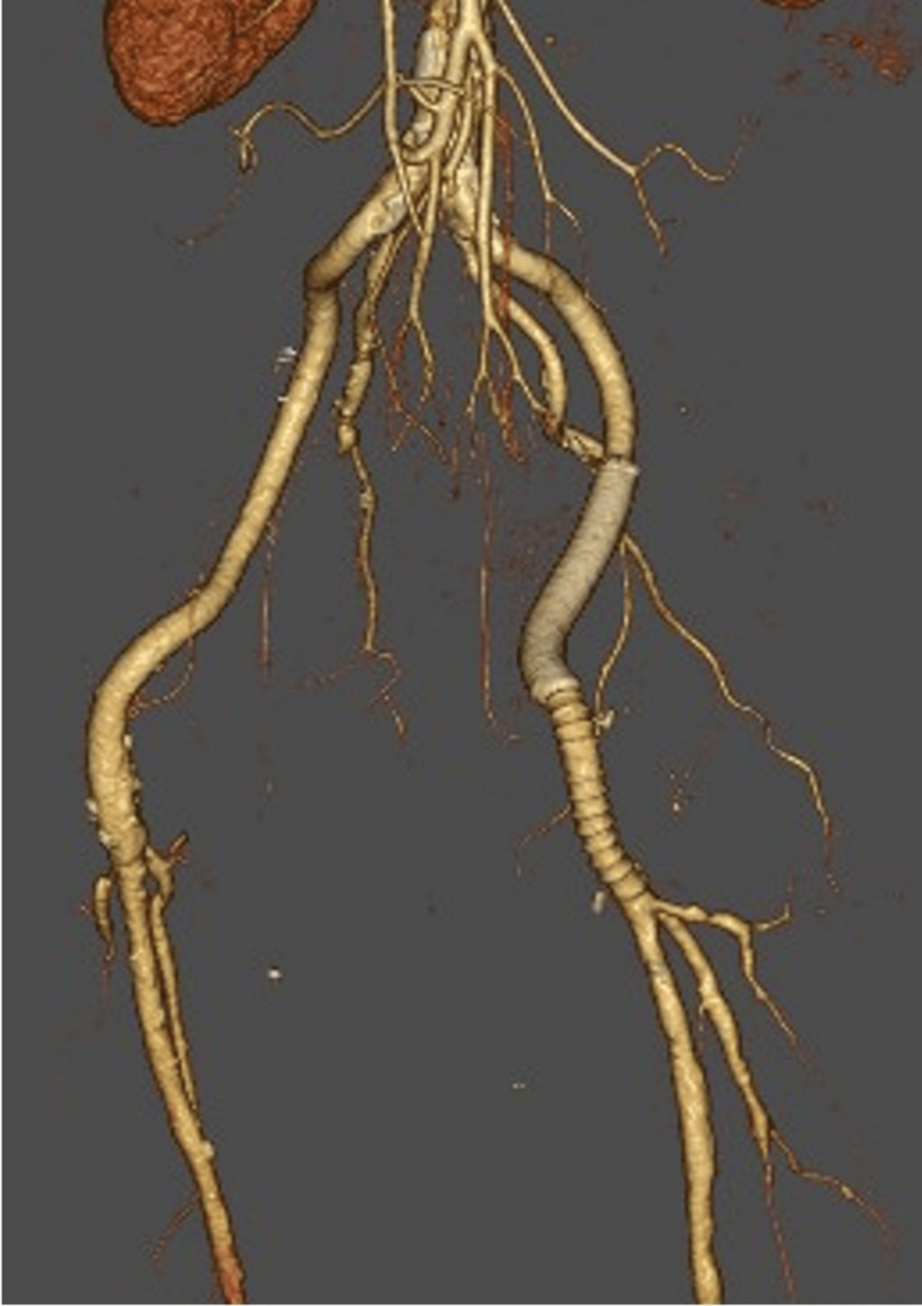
3D CT imaging demonstrates that the stent graft and artificial vessels remain intact and do not exhibit any signs of failure 3D: three-dimensional, CT: computed tomography
